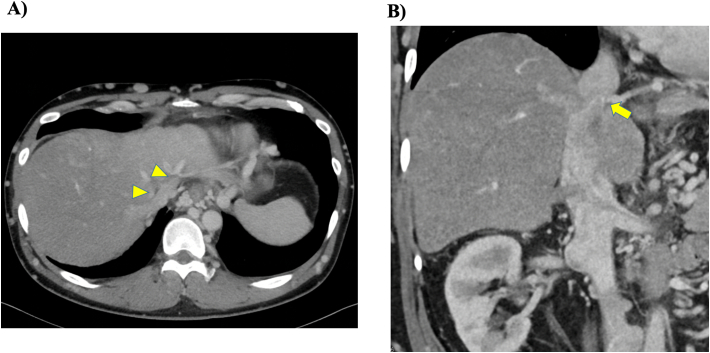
Preoperative contrast-enhanced computed tomography. Stenosis of the IVC just above the confluence of hepatic veins. Heterogenous enhancement of parenchyma. Development of esophageal varices. Allow shows the stenotic lesion of the IVC. Allow heads show the occluded middle hepatic vein and left hepatic vein.
